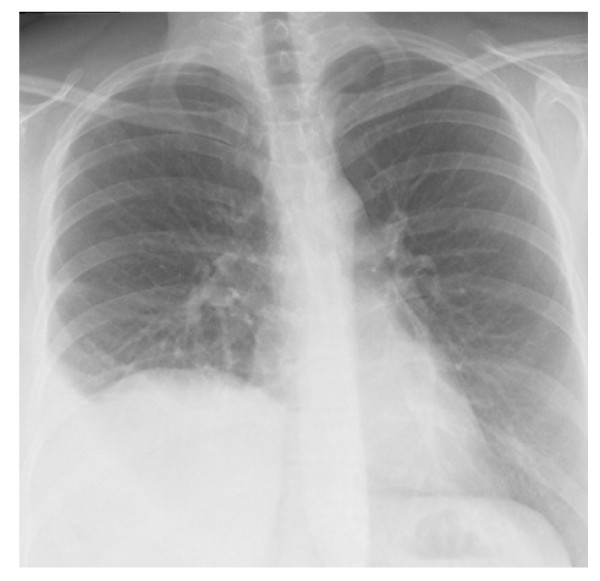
Preoperative chest X-ray showing a right-sided pleural effusion and an elevated right hemidiaphragm.
